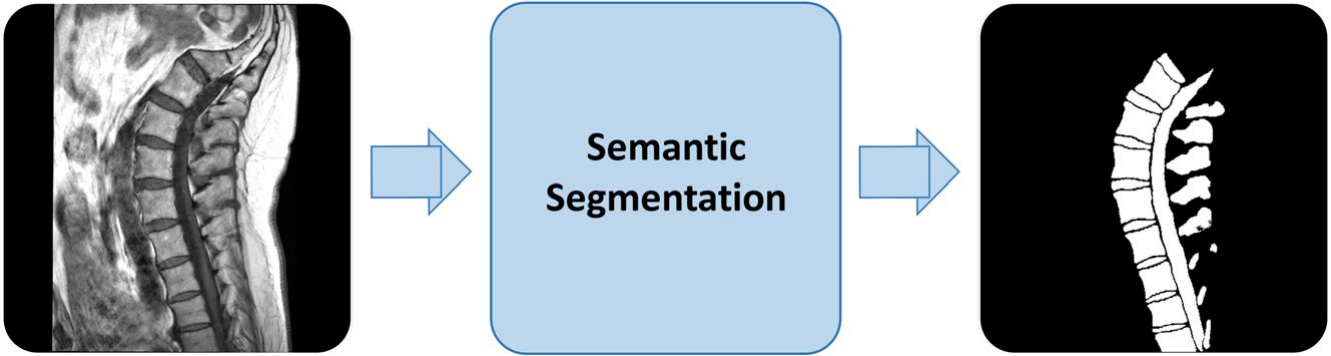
Overview of the semantic segmentation process applied to a sagittal T1-weighted spinal MRI image. Vertebrae, IVDs, and the spinal canal are identified as foreground, while all other regions are classified as background. The output is a binary segmentation map highlighting these anatomical structures.
